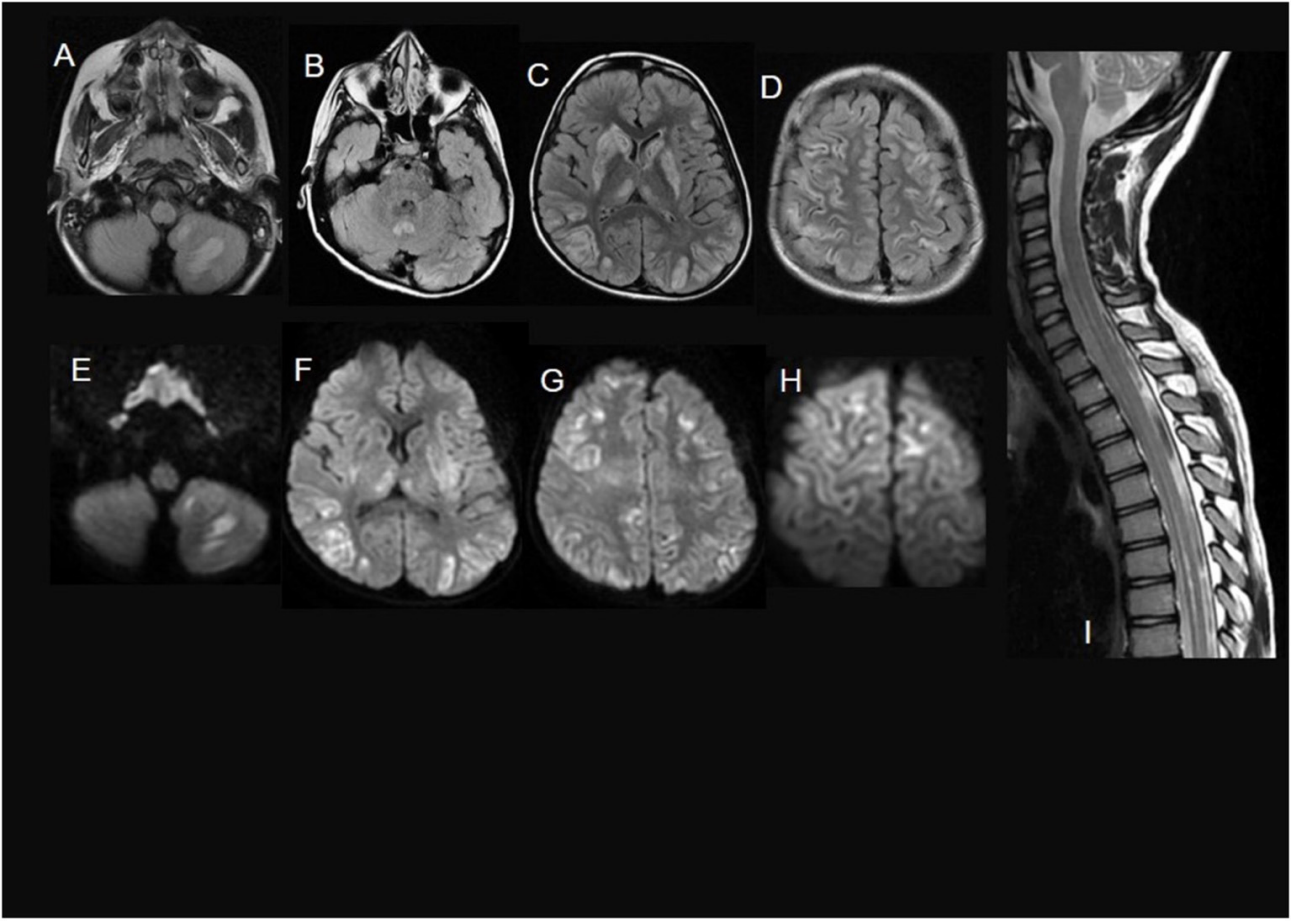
A 7-year-old child with Biotin thiamine responsive basal ganglia disease due to *SLC19A3* mutation. (A–D) axial FLAIR sections show multiple bilateral infra and supratentorial FLAIR hyperintense lesions involving the striatum, the medial thalami, the vermis, and the cortical/juxtacortical fronto-parietal and temporal areas. In (E–H), diffusion restriction in many of these lesions is noted. Sagittal T2WI (I) shows an intramedullary T2 hyperintense lesion along with the lower cervical and dorsal cord till level opposite to 10th thoracic vertebra.
